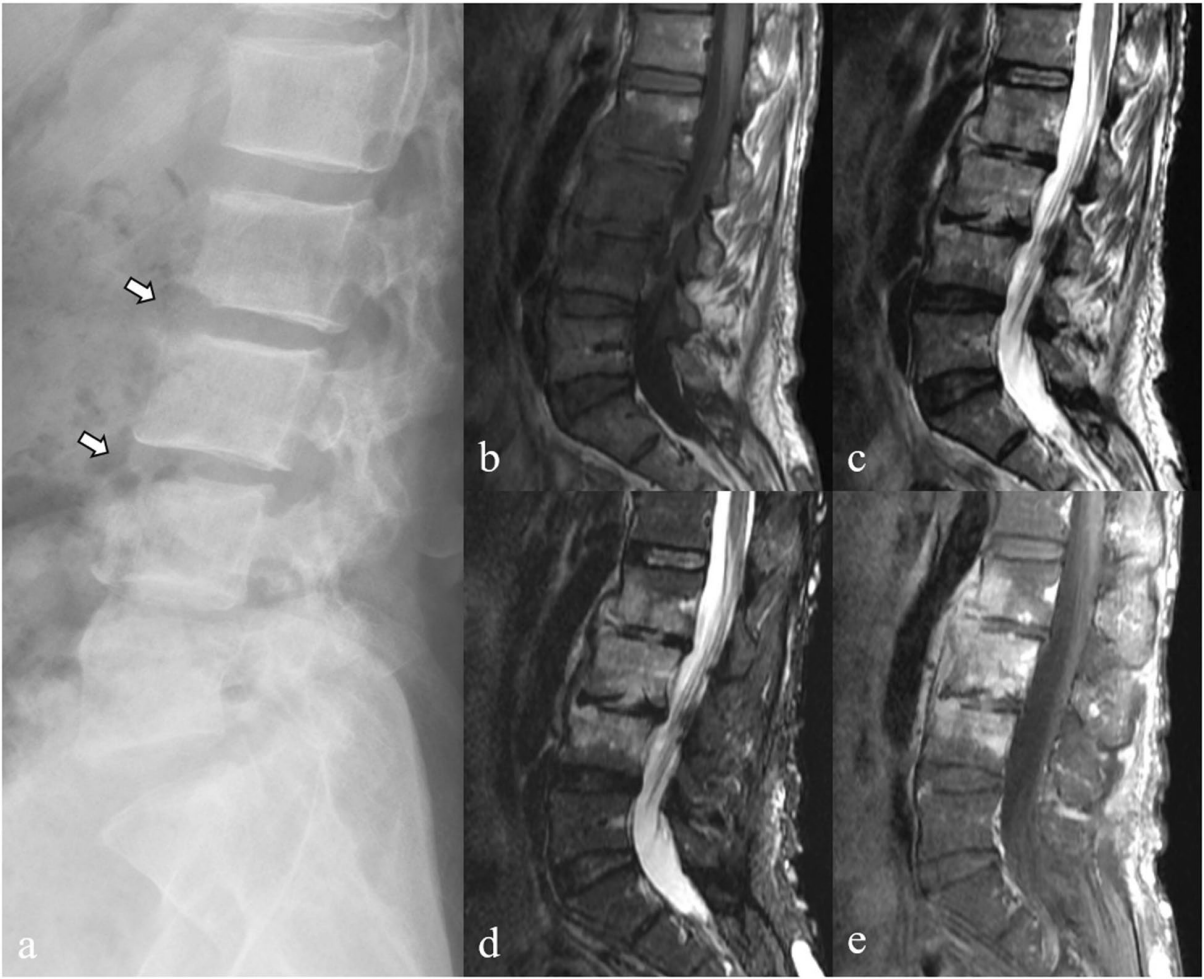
A case of diffuse brucellar spondylodiscitis in a 56-year-old female patient with underlying type I neurofibromatosis. A lateral plain radiograph (a) shows erosion of the anterior opposing endplates at L2–L3 to L3–L4 levels (arrows) with a few small anterior osteophytes. Sagittal T1-weighted (b), T2-weighted (c), short tau inversion recovery (STIR) (d), and fat-suppressed contrast-enhanced T1-weighted (e) images show hypointense T1-weighted and hyperintense T2-weighted/STIR signals with enhancement of L2–L4 vertebral bodies and intervening discs, which reveal the absence of nuclear clefts
